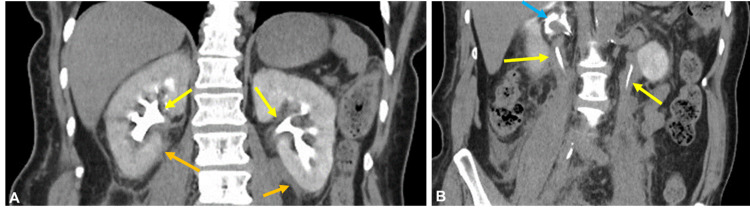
Reconstructed coronal contrast-enhanced CT images of the abdomen and pelvis obtained in the renal excretory phase with consecutive images through the kidneys (A) and ureters (B) again demonstrate the hyperdense fluid likely consisting of mixed hemorrhagic products and urine (orange arrows), with mural thickening involving the renal pelves bilaterally (yellow arrows). There is a lack of dilation of the collecting system despite narrowed lumen of the collecting system. Extravasated retroperitoneal contrast is again seen surrounding the right renal vein (blue arrow).
